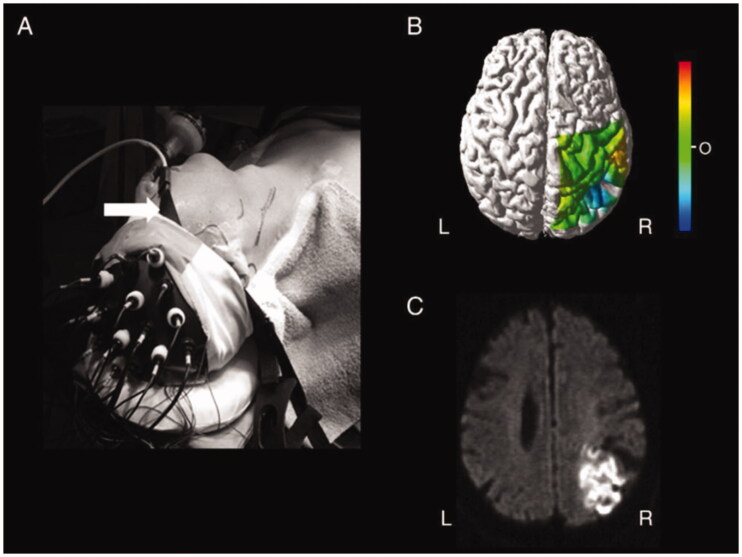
Multichannel NIRS optical topography perioperative stroke detection. (A) The placement of the optodes (OMM 2000) and the sensor (INVOS 3110 A) during surgery. White arrow indicates the sensor of the INVOS 3110 A. (B) Optical topographic maps demonstrate decreases in Oxy-Hb in the watershed zone between the MCA and PCA on the right side during cross-clamping of the ICA. The maps were overlaid on anatomical MRI surface images. C: Diffusion-weighted MRI shows high intensity regions corresponding to the area associated with the decrease of Oxy-Hb. Abbreviations: ICA, internal carotid artery; MCA, middle cerebral artery; Oxy-Hb, oxyhaemoglobin; PCA, posterior cerebral artery; OT, optical topography. [Reprinted from Nakamura et al. [59] with permission from Elsevier].
